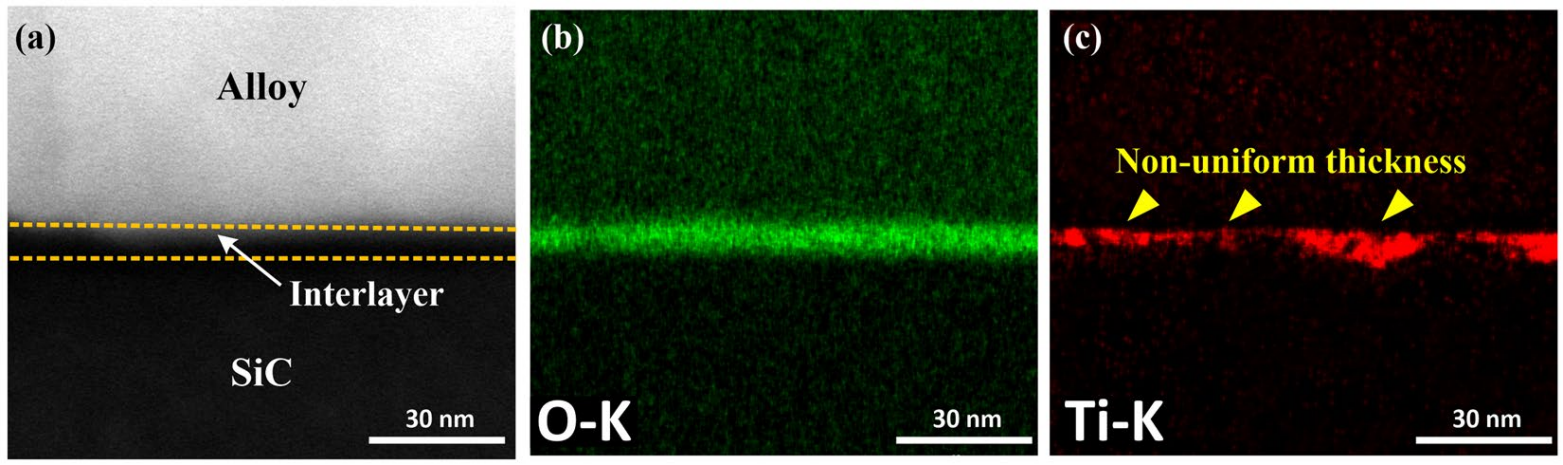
(a) HADDF image of the interface soldered with ultrasonic action for 0.1 s and constant temperature treatment for 5 min; (c,d) the corresponding STEM-EDS maps of O and Ti, respectively.
